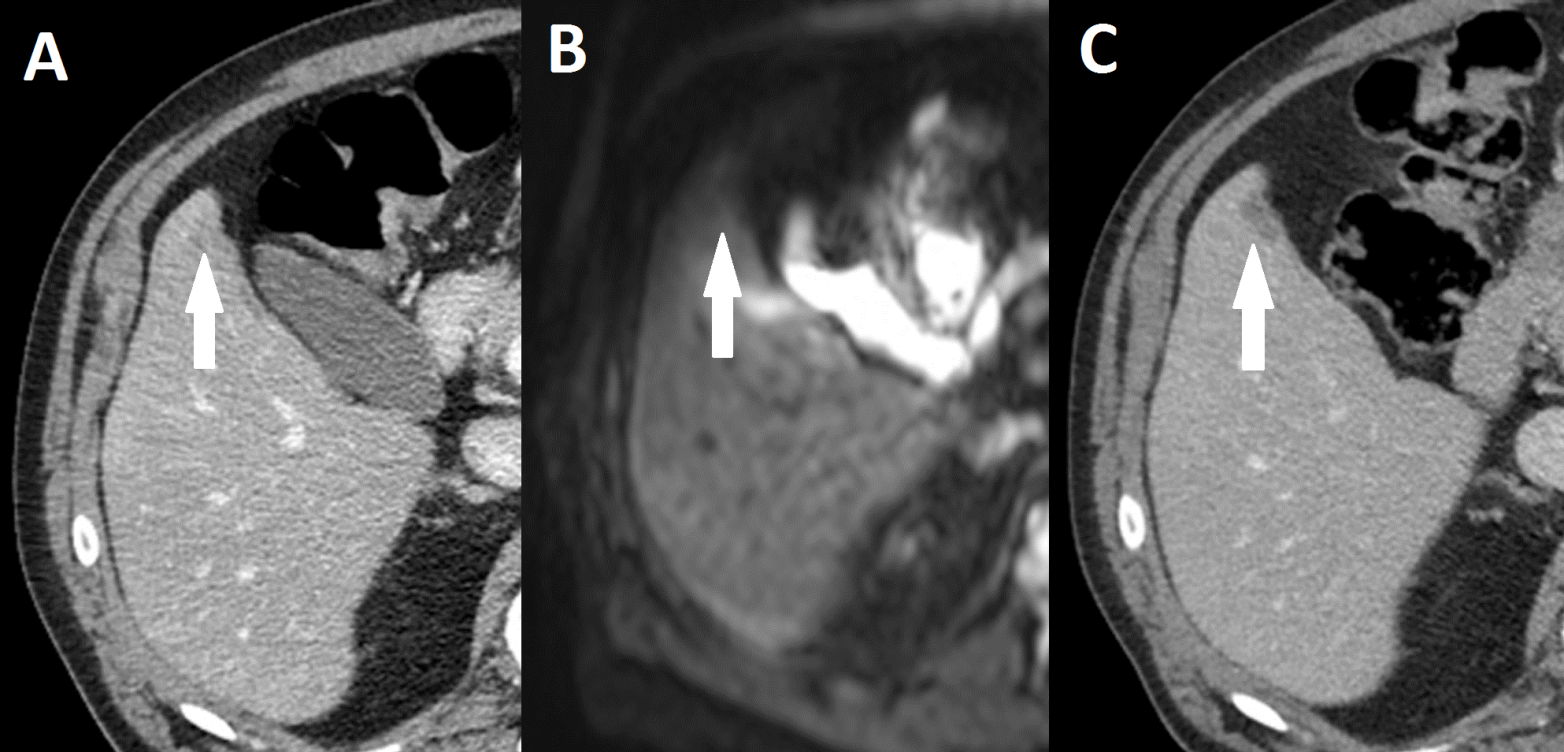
Missed liver metastasis on CT and diffusion weighted MR. (A) Initial CT, (B) Diffusion weighted MR, (C) subsequent CT after 5 months of neoadjuvant therapy. Broken arrow: Metastasis missed on primary CT and diffusion weighted MR.
